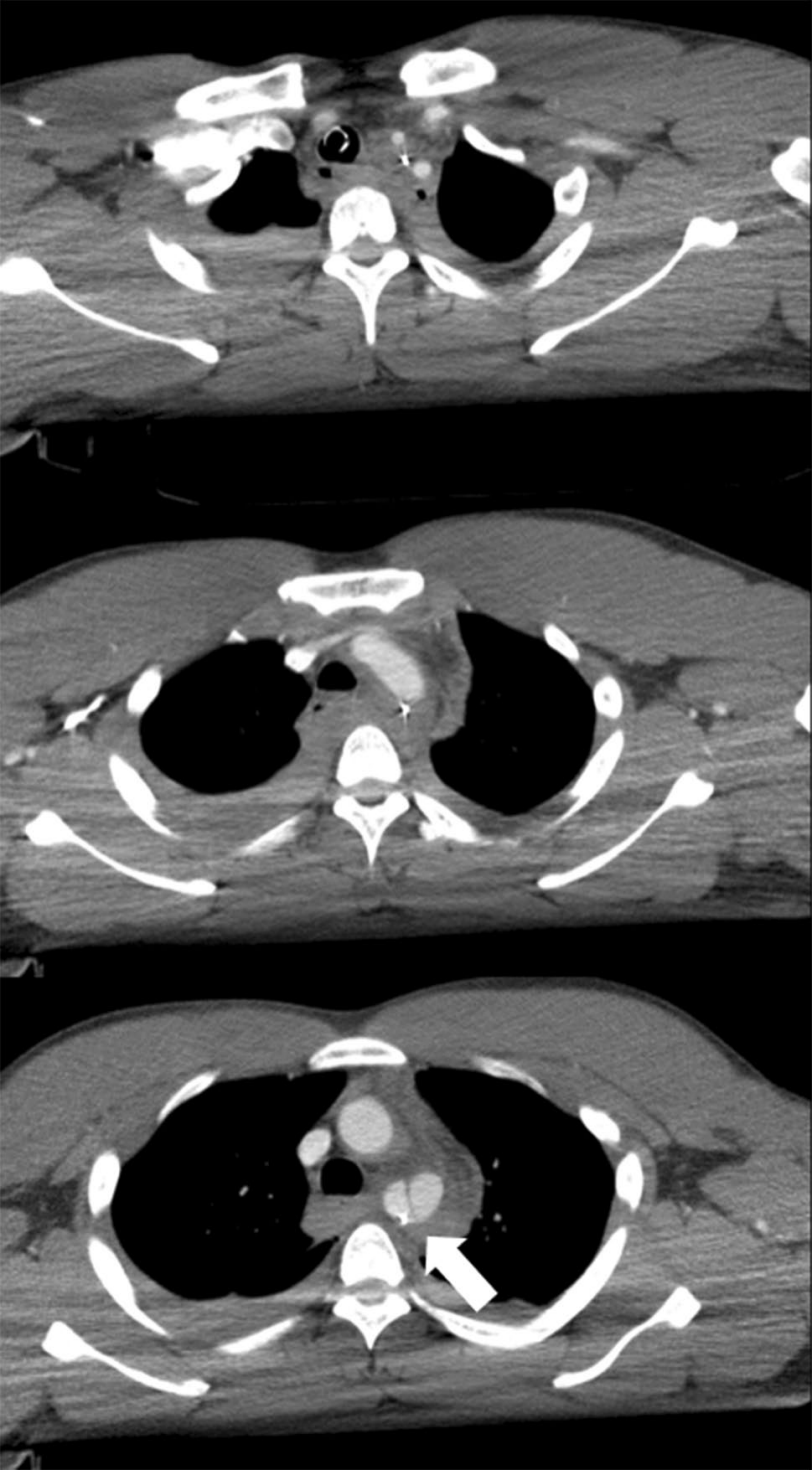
Thoracic contrast-enhancement computed tomography in a patient with multiple traumas showing a complication of the guidewire migration into the arterial subintima (arrow). Resuscitative endovascular balloon occlusion of the aorta (REBOA) is contraindicated in a thoracic aortic injury, but the injury was not expected in the REBOA procedure in this case
